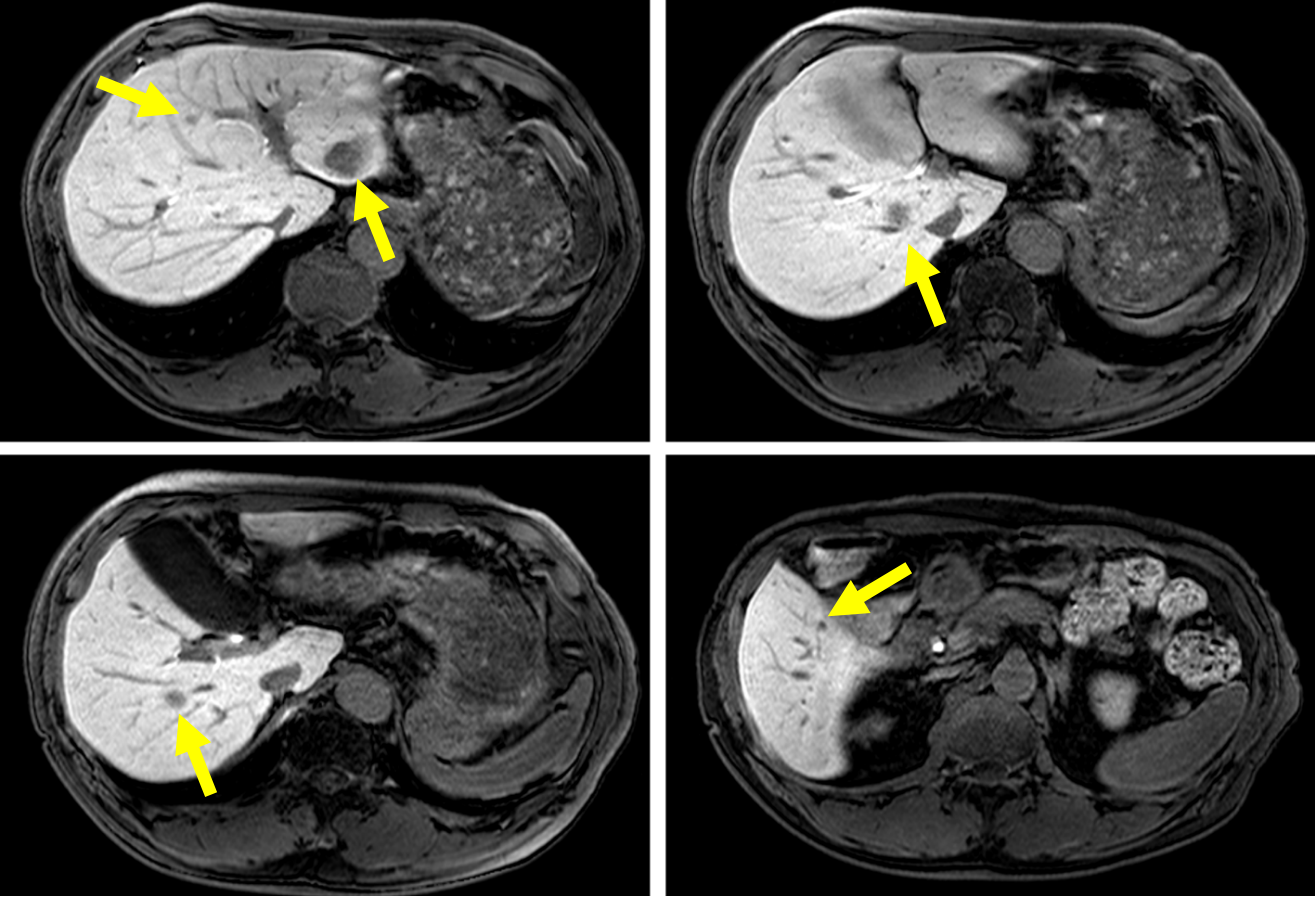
Gadoxetic acid (Gd-EOB-DTPA)-enhanced MRI for detection of liver metastases showed multiple liver metastases before chemotherapy
